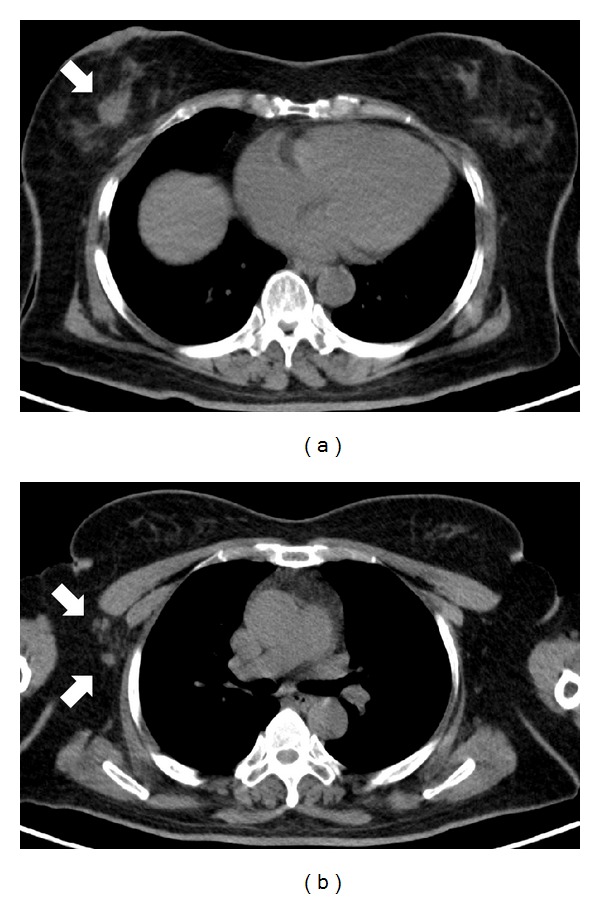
Incidental right breast mass (a) arrow, 2.7 × 1.7 cm in size) and several right axillary lymph nodes (b) arrows, the largest one is 1.0 × 0.8 cm in size) in a 59-year-old female with history of endometrial cancer were found by followup unenhanced CT of ^18^F-fluoride PET/CT. The biopsy for the right breast tumor confirmed the presence of invasive ductal carcinoma.
